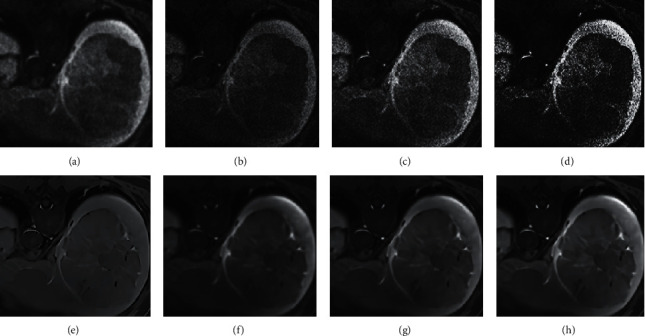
MRI images of a PHC patient before and after processing by the bias field correction algorithm.
